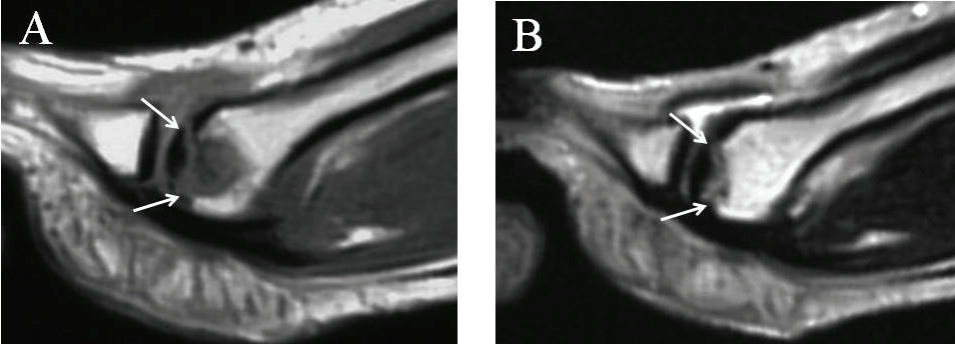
Magnetic resonance imaging (MRI) studies of the metatarsophalangeal joint of the right second toe. (A) A low-intensity zone was detected within the second metatarsal head in the sagittal plane of the T1-weighted MRI scan. (B) A high-intensity zone was detected at the subchondral bone in the sagittal plane of the T2-weighted MRI scan.
